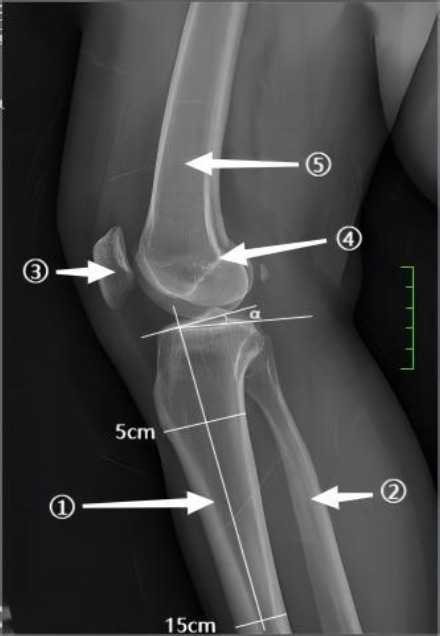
X-ray lateral view of the knee joint. The figure shows: (1) Tibia; (2) Fibula; (3) Patella; (4) Blumensaat's line; (5) Femur; ∠α (the posterior tibial slope angle) =10.7°. (Source: Radiology Department, Baise People's Hospital, Guangxi, China).
